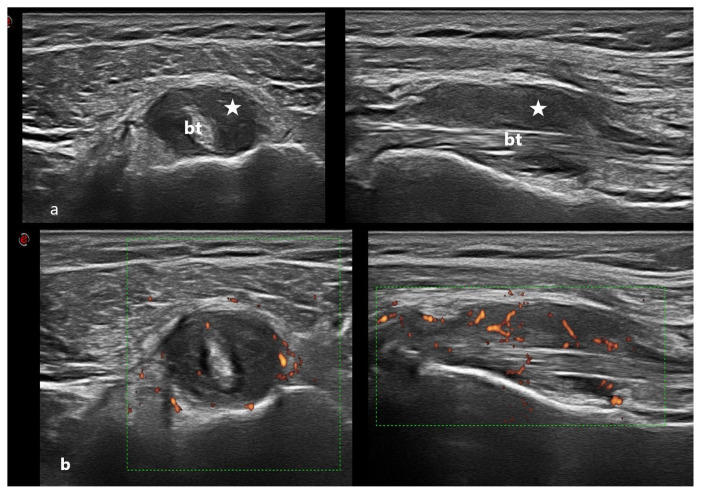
Gray scale (a) and Doppler scale (b) US performed on a shoulder with PMR diagnosis, the patient has edema and an anechoic effusion area around the shoulder with a thickened biceps tendon in both planes (axial and longitudinal). Stars indicate synovial hypertrophy, and bt shows the biceps tendon.
